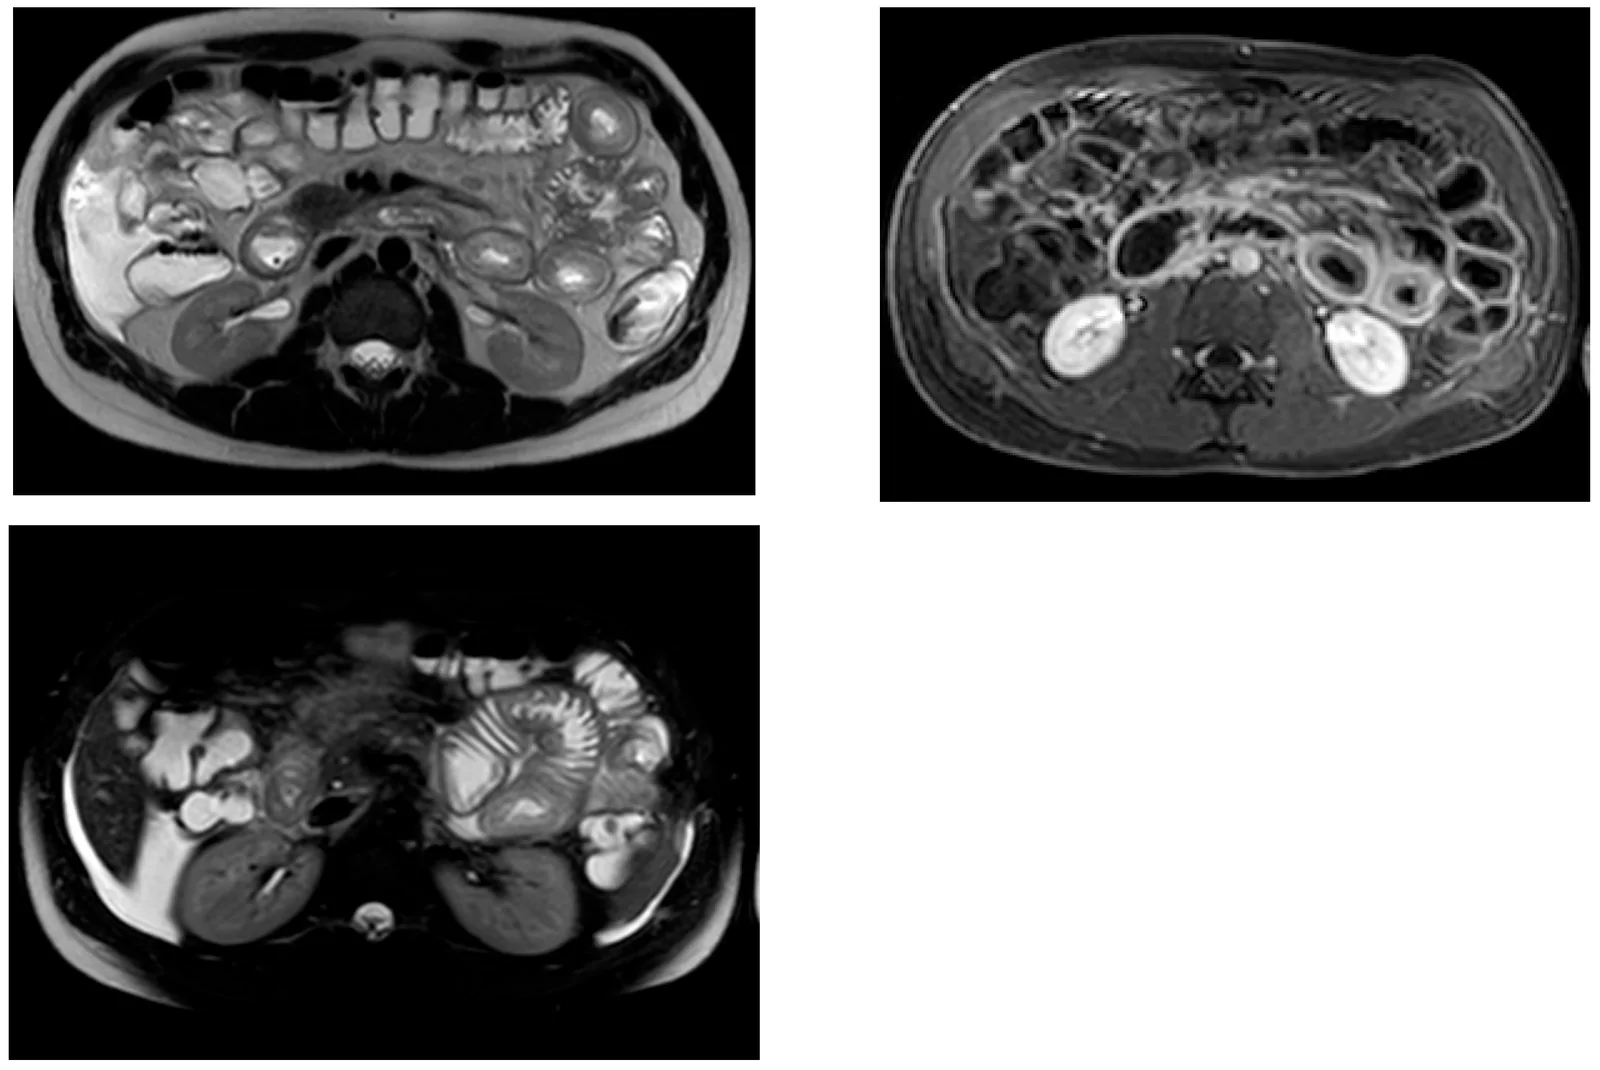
Contrast-enhanced abdominal–pelvic MRI. T2 and post-contrast T1-weighted sequence in the axial plane: thickened jejunal loops and intraperitoneal fluid.
